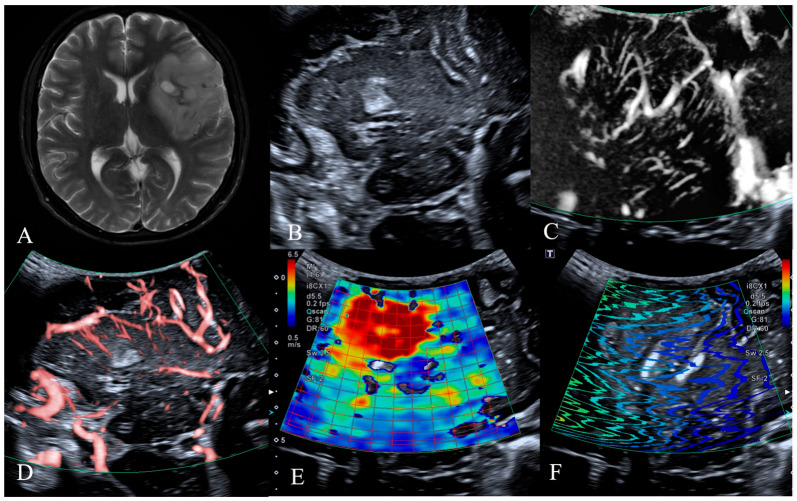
MRI, B-mode, SMI and SWE of LGG in a 54-year-old man. The postoperative pathology was oligodendroglioma, IDH mutant type. (A) Preoperative T2-weighted magnetic resonance imaging revealed a large irregular mass in the right fronto-temporo-insular region, exhibiting heterogeneous internal signal intensity with small areas of calcification identified. (B) B-mode ultrasonography revealed an irregularly shaped, ill-defined hypoechoic mass without significant peritumoral edema, with internal calcification identified. (C,D) SMI mode, (C) is mSMI mode, (D) is cSMI mode. The vascular architecture in tumor parenchyma is straight and branching, and the vascular architecture around the tumor is normal cerebral vessels. (E,F) SWE mode. (E) Red areas indicate higher stiffness, with the intratumoral region exhibiting significantly increased stiffness compared to the surrounding peritumoral tissue. The mean Young‘s modulus value obtained from repeated measurements of tumor tissue was 35.8 kPa. (F) The shear wave propagation map demonstrates that shear waves propagate smoothly within the tumor, with high reliability of data acquisition.
